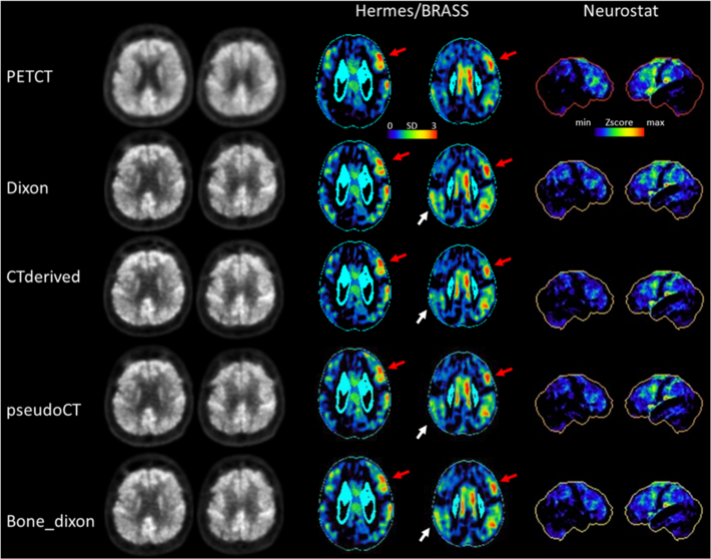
Patient example. Sixty-five-year-old women with clinical and imaging-based diagnosis of corticobasal degeneration. Relative FDG uptake was severely impaired in the left frontal regions (*red arrows*). Additionally, there was some degree of relative bilateral uptake deficiency in parietal areas which was more pronounced in PET_Dixon_ but also apparent in PET_BoneDixon_ as compared to PET_CTderived_ (*white arrows*), resulting in an imaging diagnosis of Alzheimer’s disease in PET_PseudoCT/BoneDixon_ for reader 1 and in PET_Dixon/PseudoCT/BoneDixon_ for reader 2. Note: readers were blinded to the clinical diagnosis
